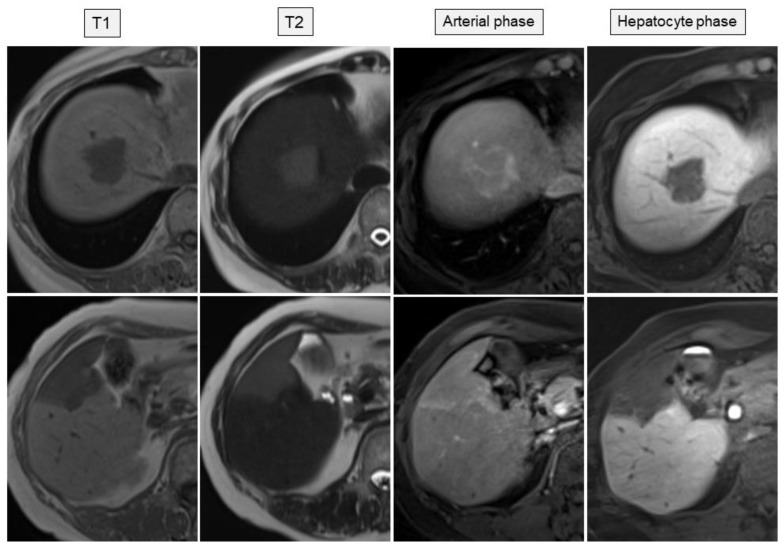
Gd-EOB-DTPA-enhanced magnetic resonance imaging visualized the lesions at segments S8 (upper panel) and S5 (lower panel) in the liver as low-intensity lesions in T1-weighted images, high-intensity lesions in T2-weighted images, ring-enhancement pattern lesions in the arterial phase, and mainly low intensity lesions in the hepatobiliary phase.
